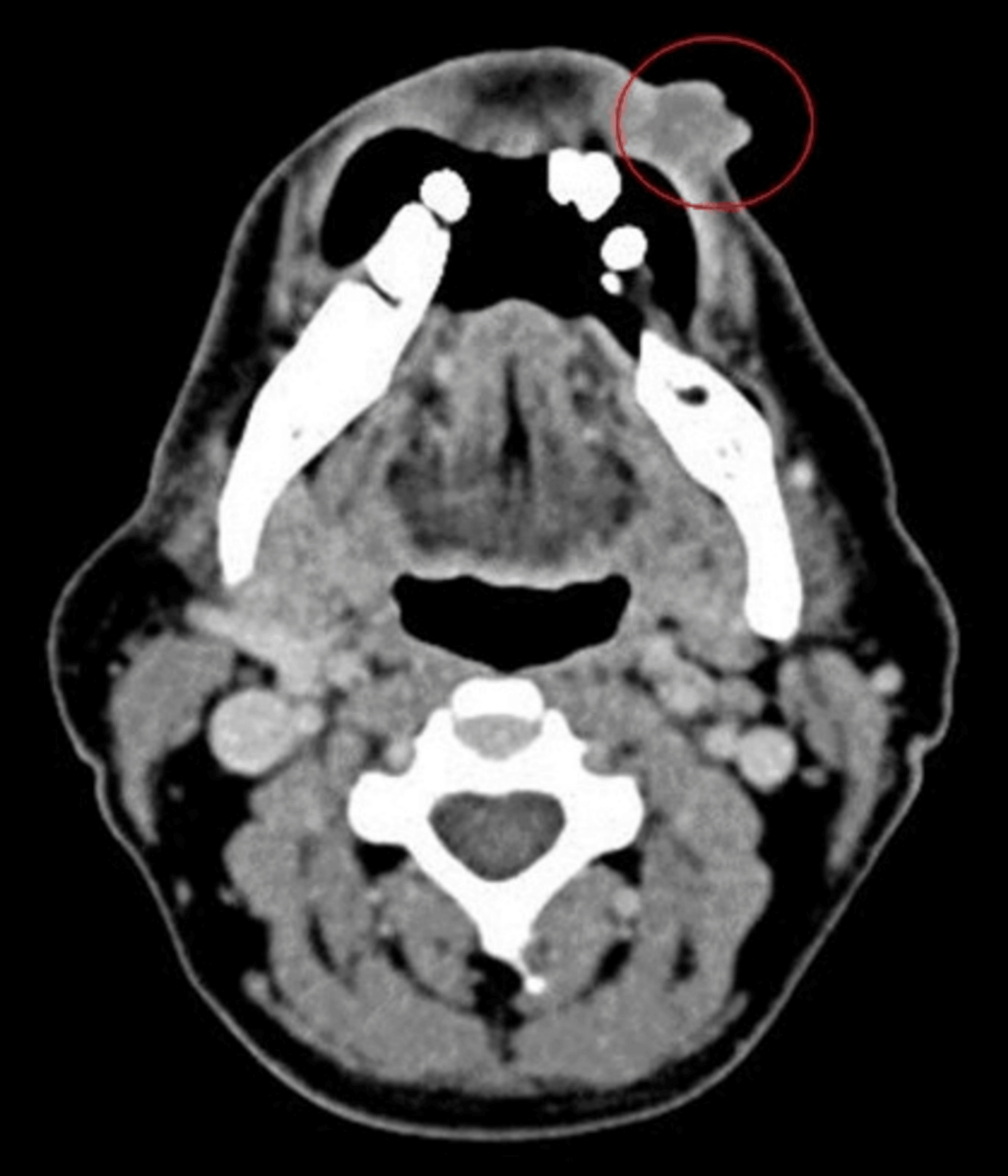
Cross-sectional CECT image CECT: contrast-enhanced computed tomography
